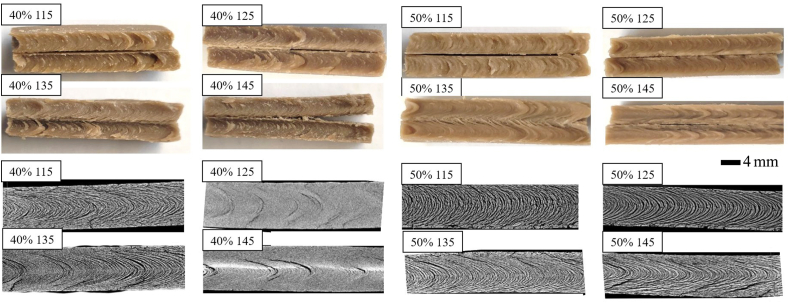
Visual appearance of the fracture profile (top) and micro-computed tomography images (bottom) of dry-fractionated mung bean protein concentrate extruded at different temperatures and moisture contents. The flow direction is from the left to the right.
